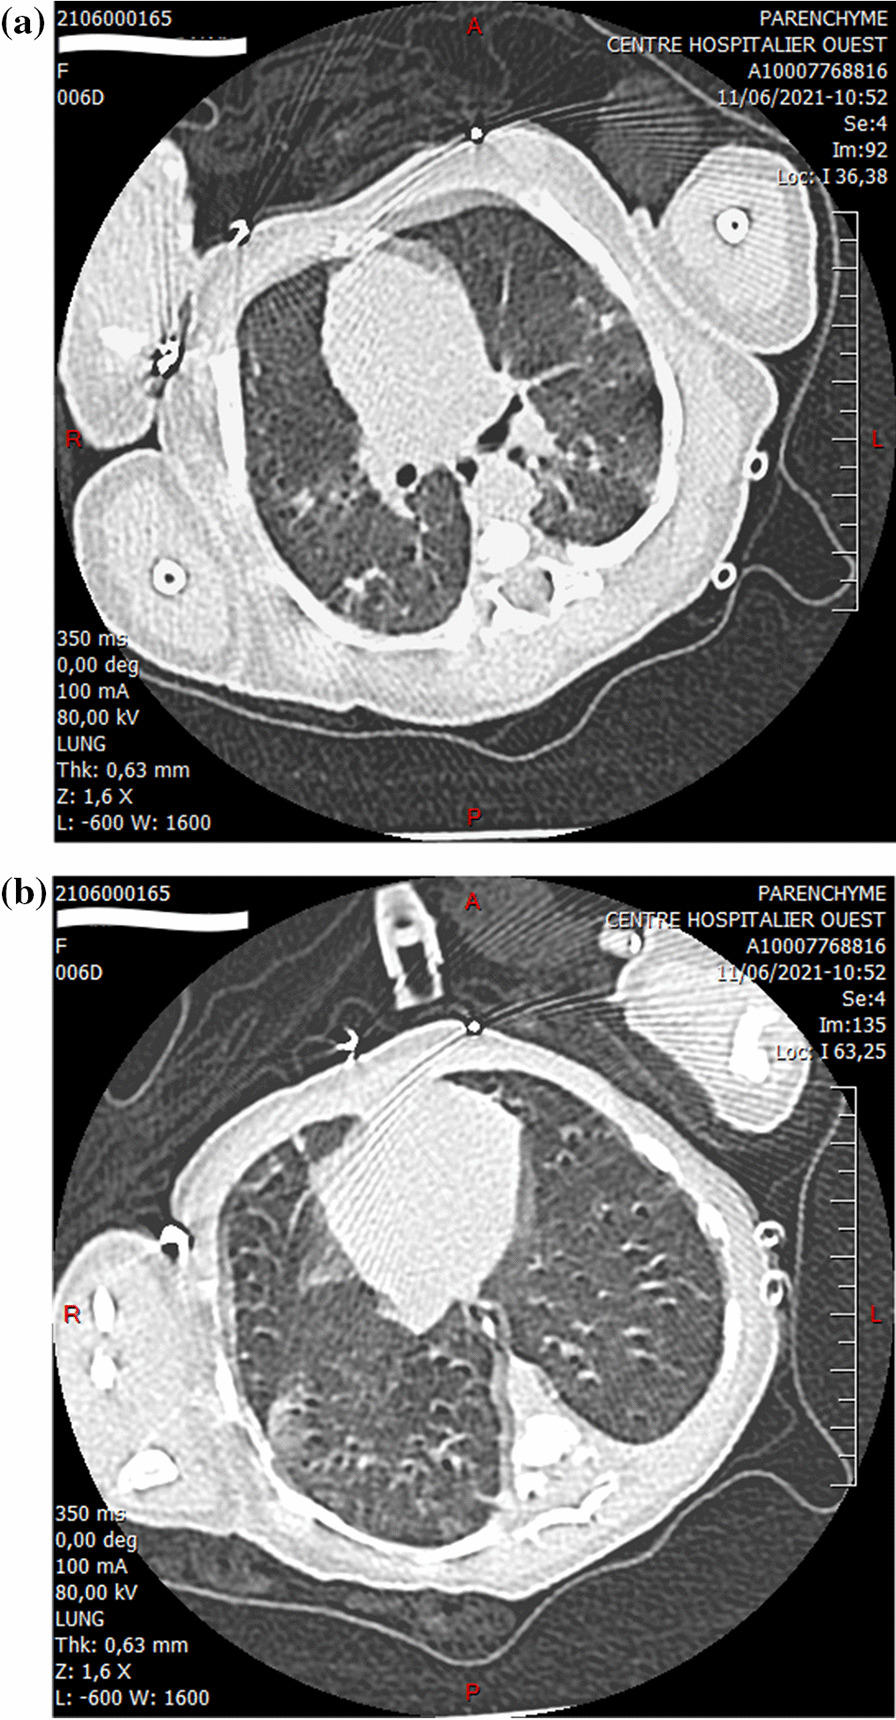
a Computed tomography scan showing bilateral parenchymatous lesions (10–20%), in ground glass, compatible with a coronavirus disease-type infection. b This figure also shows ground glass pictures
